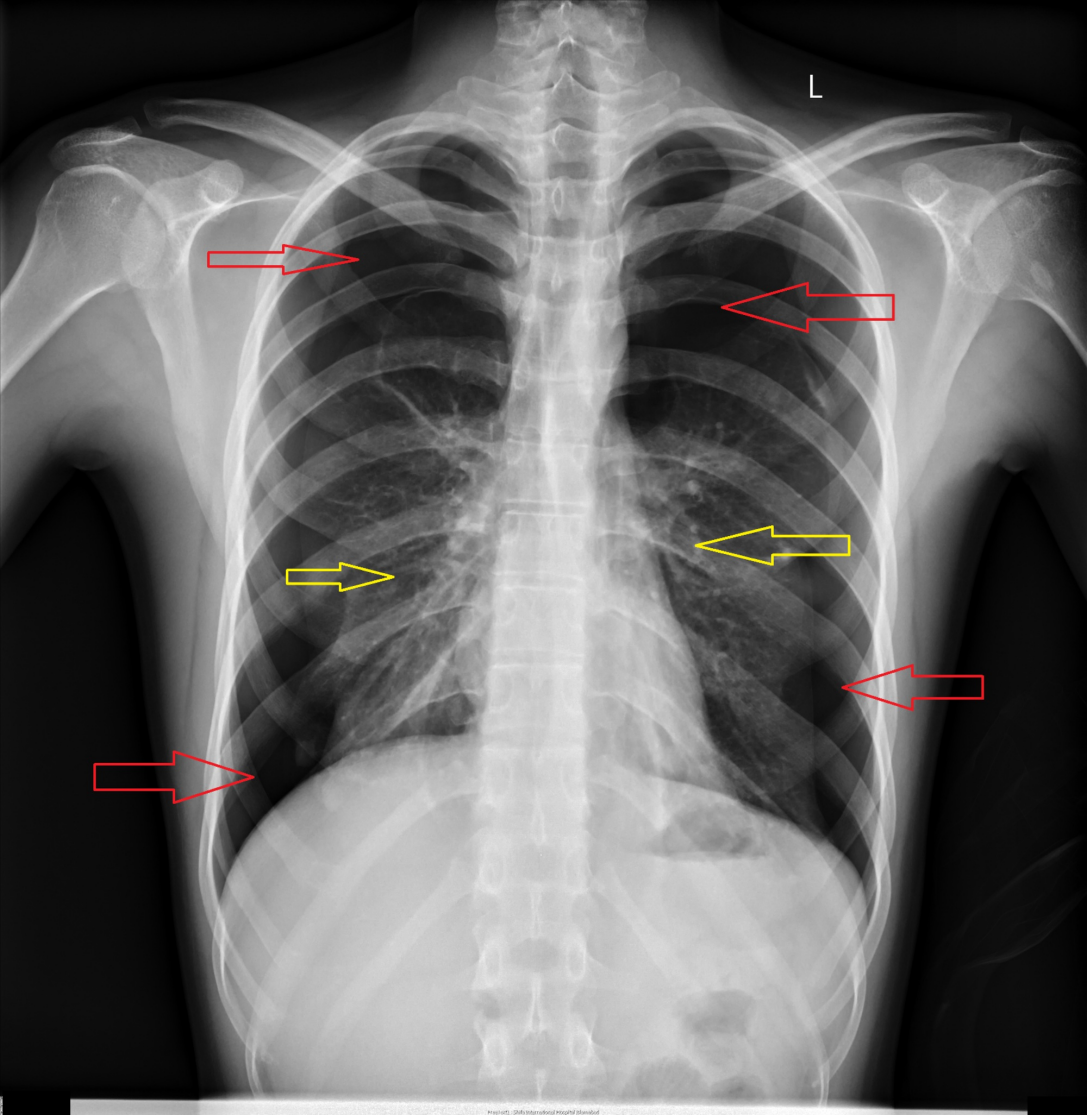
Chest X-ray showing bilateral pneumothorax. Red arrows point toward highly radiolucent areas in the thorax demonstrating bilateral pneumothorax. Yellow arrows point toward medially displaced lung parenchyma due to bilateral pneumothorax.
